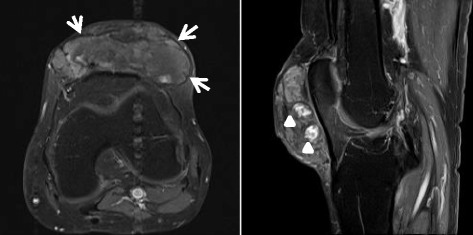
Gadolinium-enhanced T1-weighted magnetic resonance imaging Knee gadolinium-enhanced T1-weighted MRI showed a low-intensity area in the outer regions (*arrows*) and a heterogeneously enhanced area in the central region (*arrow heads*)
